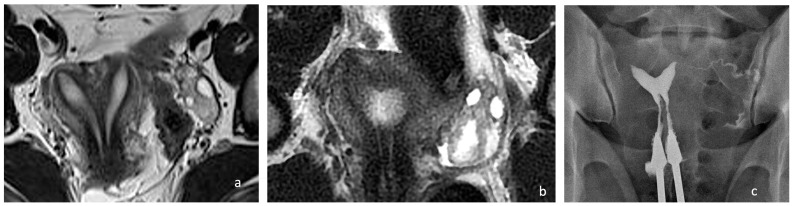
Preoperative and postoperative examination of case 1. (a) MRI T2, WI, confirmed the presence of a Müllerian anomaly and identified it as a complete septate uterus, duplicated cervix, and longitudinal vaginal septum (Class U2bC2V1 of the ESHRE/ESGE classification). Additionally, it provided information regarding the septum’s thickness, which measured 4–6 mm. (b) Postoperative MRI showing a uterine cavity. (c) Control hysterosalpingography performed 3 months after surgery, demonstrating a single uterine cavity.
